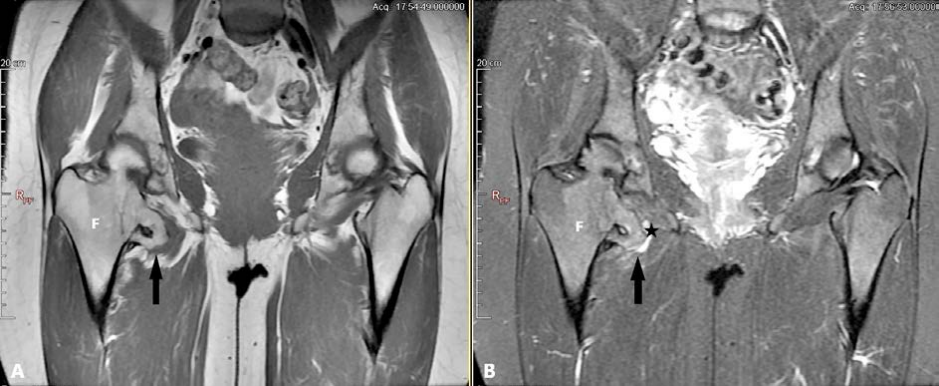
Coronal plane hip MRI images of the same patient. FSE T1W (A) and fat saturation FSE T2W (B). *Osteochondroma* (shown with black arrows) containing a 4 mm cartilage cap (asterisk) that creates *QFM* compression by narrowing the *ischiofemoral distance* on the right. Normal *ischiofemoral distance* on the left*. F: Femur.*
